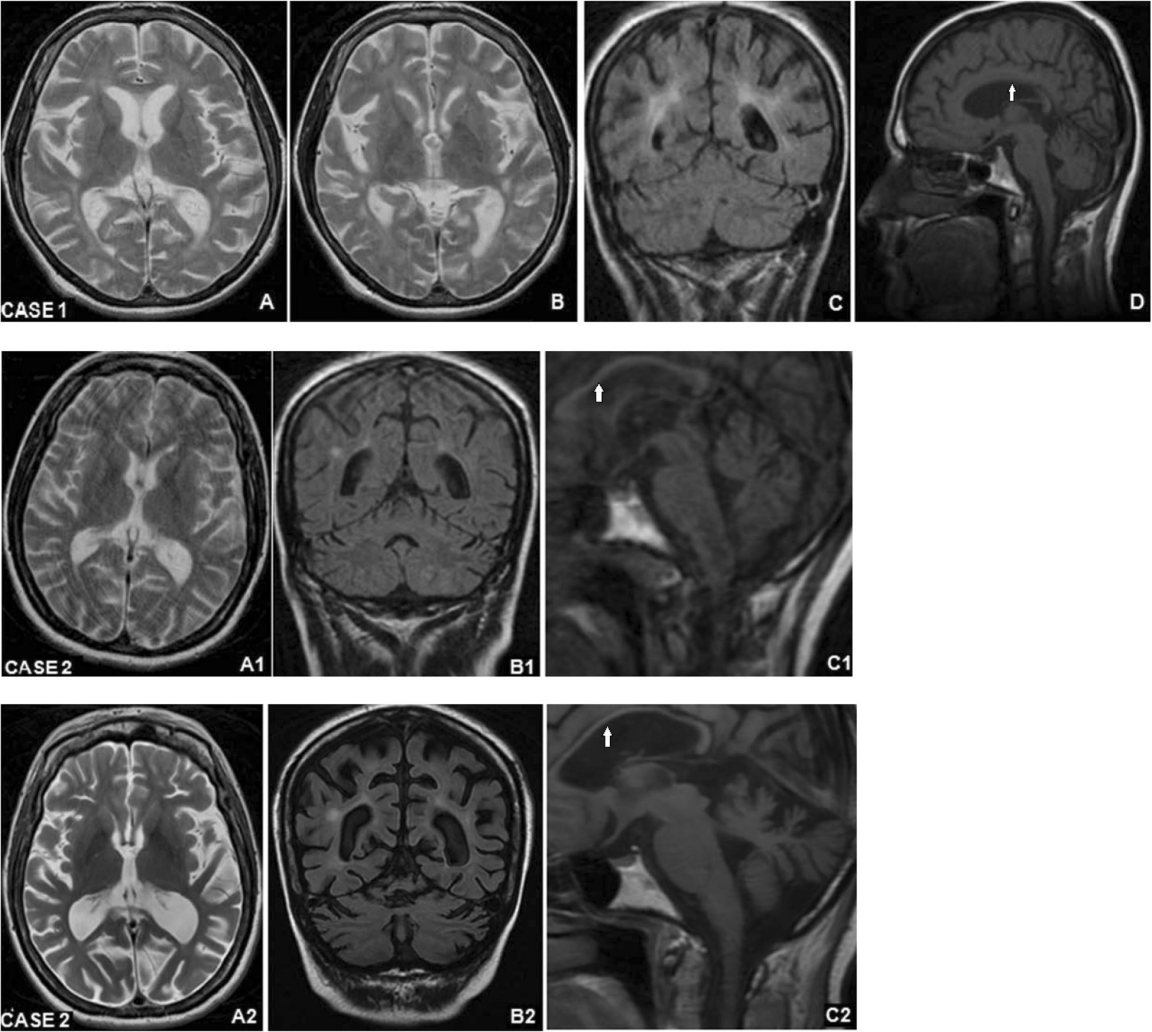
Brain MRI imaging in two patients with Kufs disease type B. *Case 1*. Axial T2-TSE (a and b), coronal T2-FLAIR (c), and sagittal T1-TSE (d) sections at onset of cognitive impairment showing cortico-subcortical atrophy, predominant in the parieto-occipital regions and the cerebellum, white matter hyperintensities and thinning of the corpus callosum (*arrow*). *Case 2*. Images at onset of cognitive impairment: axial T2-TSE (a1), coronal T2-FLAIR (b1) and sagittal T1-TSE (c1) sections displayed brain atrophy, mainly involving the cerebellum, periventricular hyperintensities and thinning of the corpus callosum (*arrow*). Follow up imaging: axial T2-TSE (a2) and coronal T2-FLAIR (b2) and sagittal T1-TSE (c2) scans taken in the same patient 9 years later
